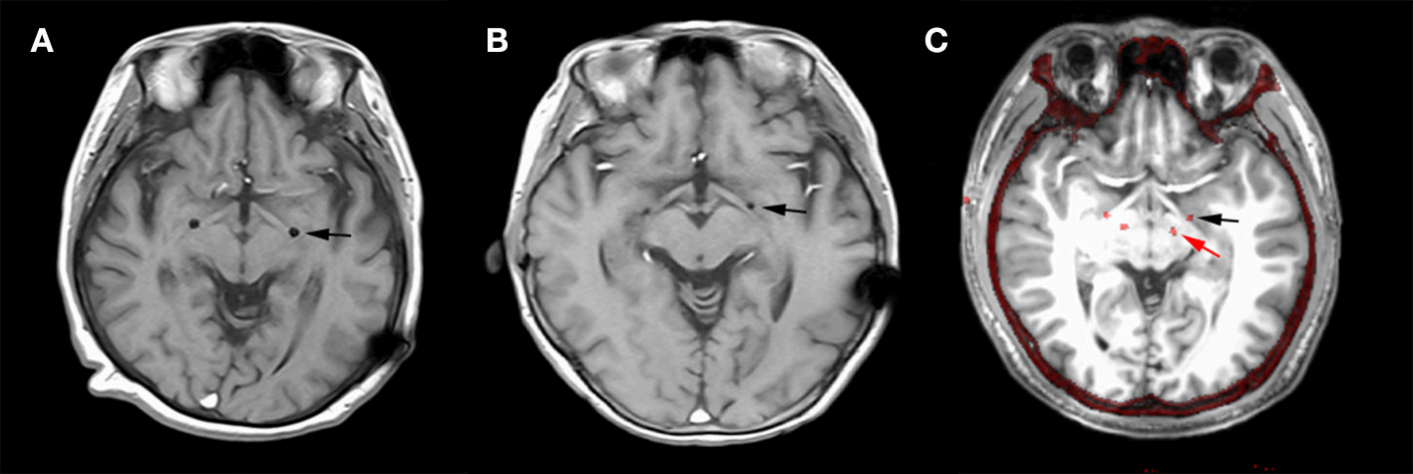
Deep brain stimulation (DBS) lead locations. Representative examples of lead locations in the left (L)-GPi of patients H2 and H6 and in both L-STN and L-GPi of patient H7 derived from post-operative MR and merged post-operative CT with pre-operative MRI. The black asterisk represents the location of GPi and the red asterisk represents the location of STN. (A) Post-operative MR showing the lead location in GPi of patient H1 on the horizontal plane. (B) Post-operative MR showing the lead location in GPi of patient H2 on the horizontal plane. (C) Merged image showing the lead locations in GPi and STN of patient H7 on the horizontal plane.
